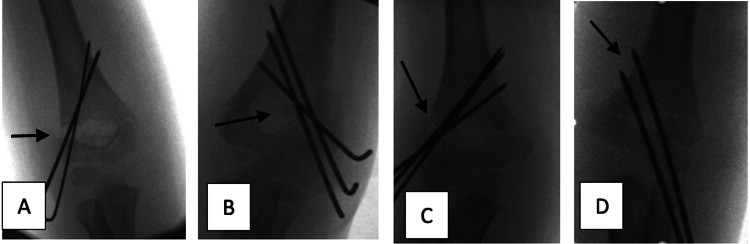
Technical errors of lateral wire fixation (A, B, C, D) Anteroposterior radiograph showing inadequate fixation (using two or three wires) of displaced supracondylar fracture (not bi-columnar, not divergent, and separation of wires <1/3 circumference of the humerus at the fracture site).
